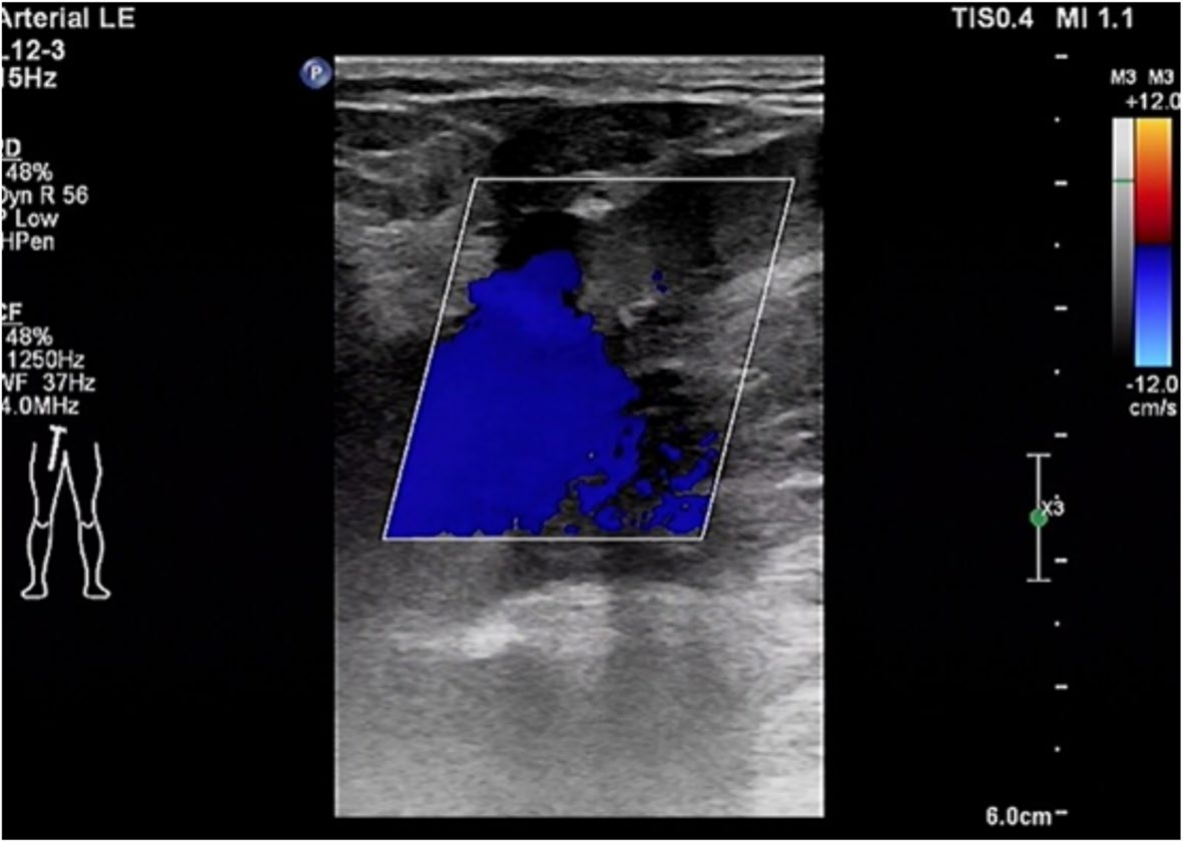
Color Doppler of the right sapheno-femoral junction showed venous stasis
